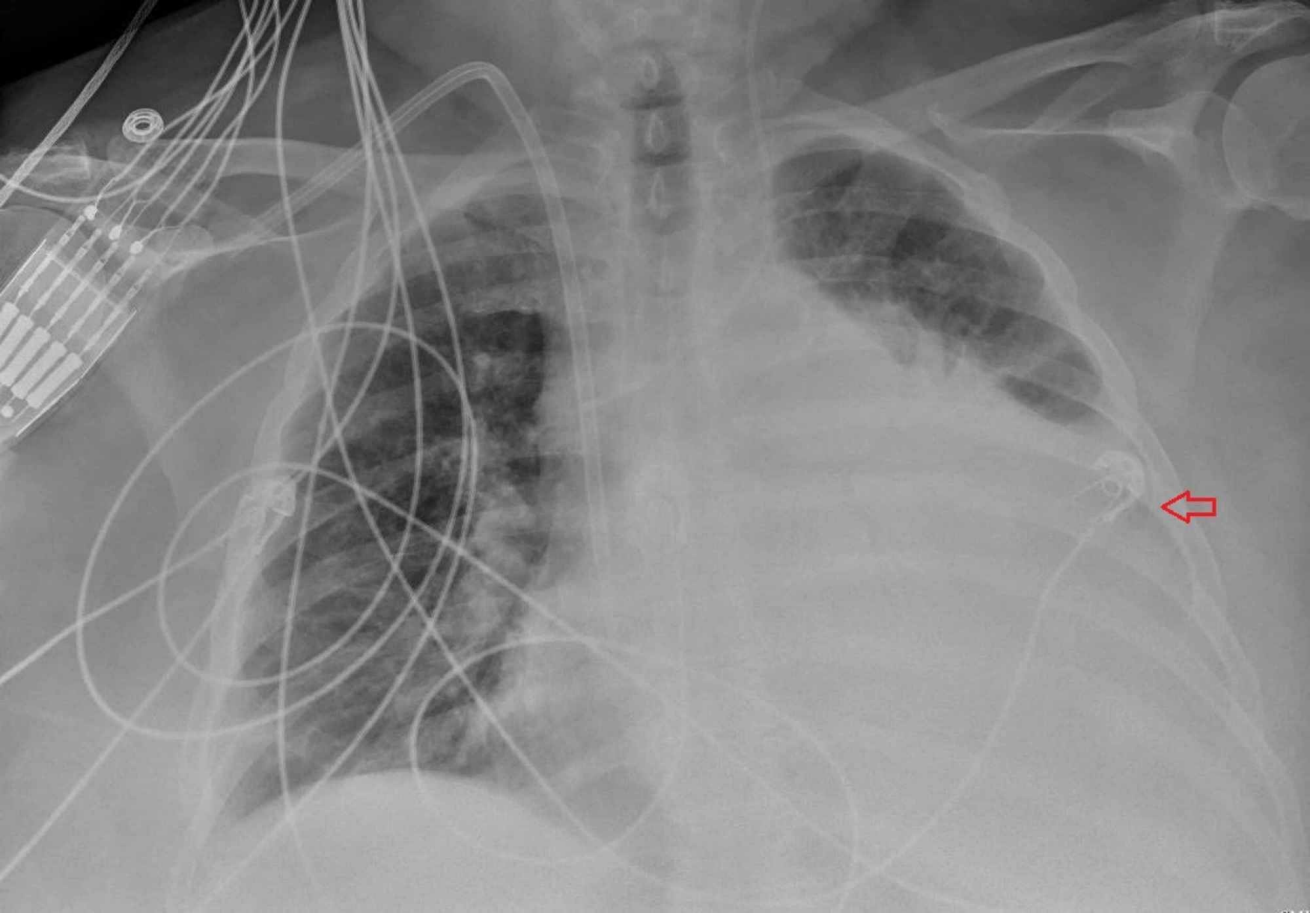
Chest X-ray showing enlargement of the cardiac silhouette and opacification of the left hemithorax (red arrow)
